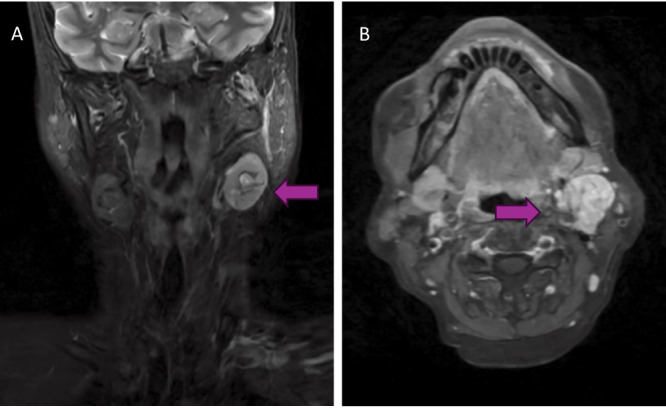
MRI neck with gadolinium contrast in coronal (A) and axial (B) demonstrating the same left sided level IIA neck mass measuring alongside a small mixed solid/cystic lymph node.
